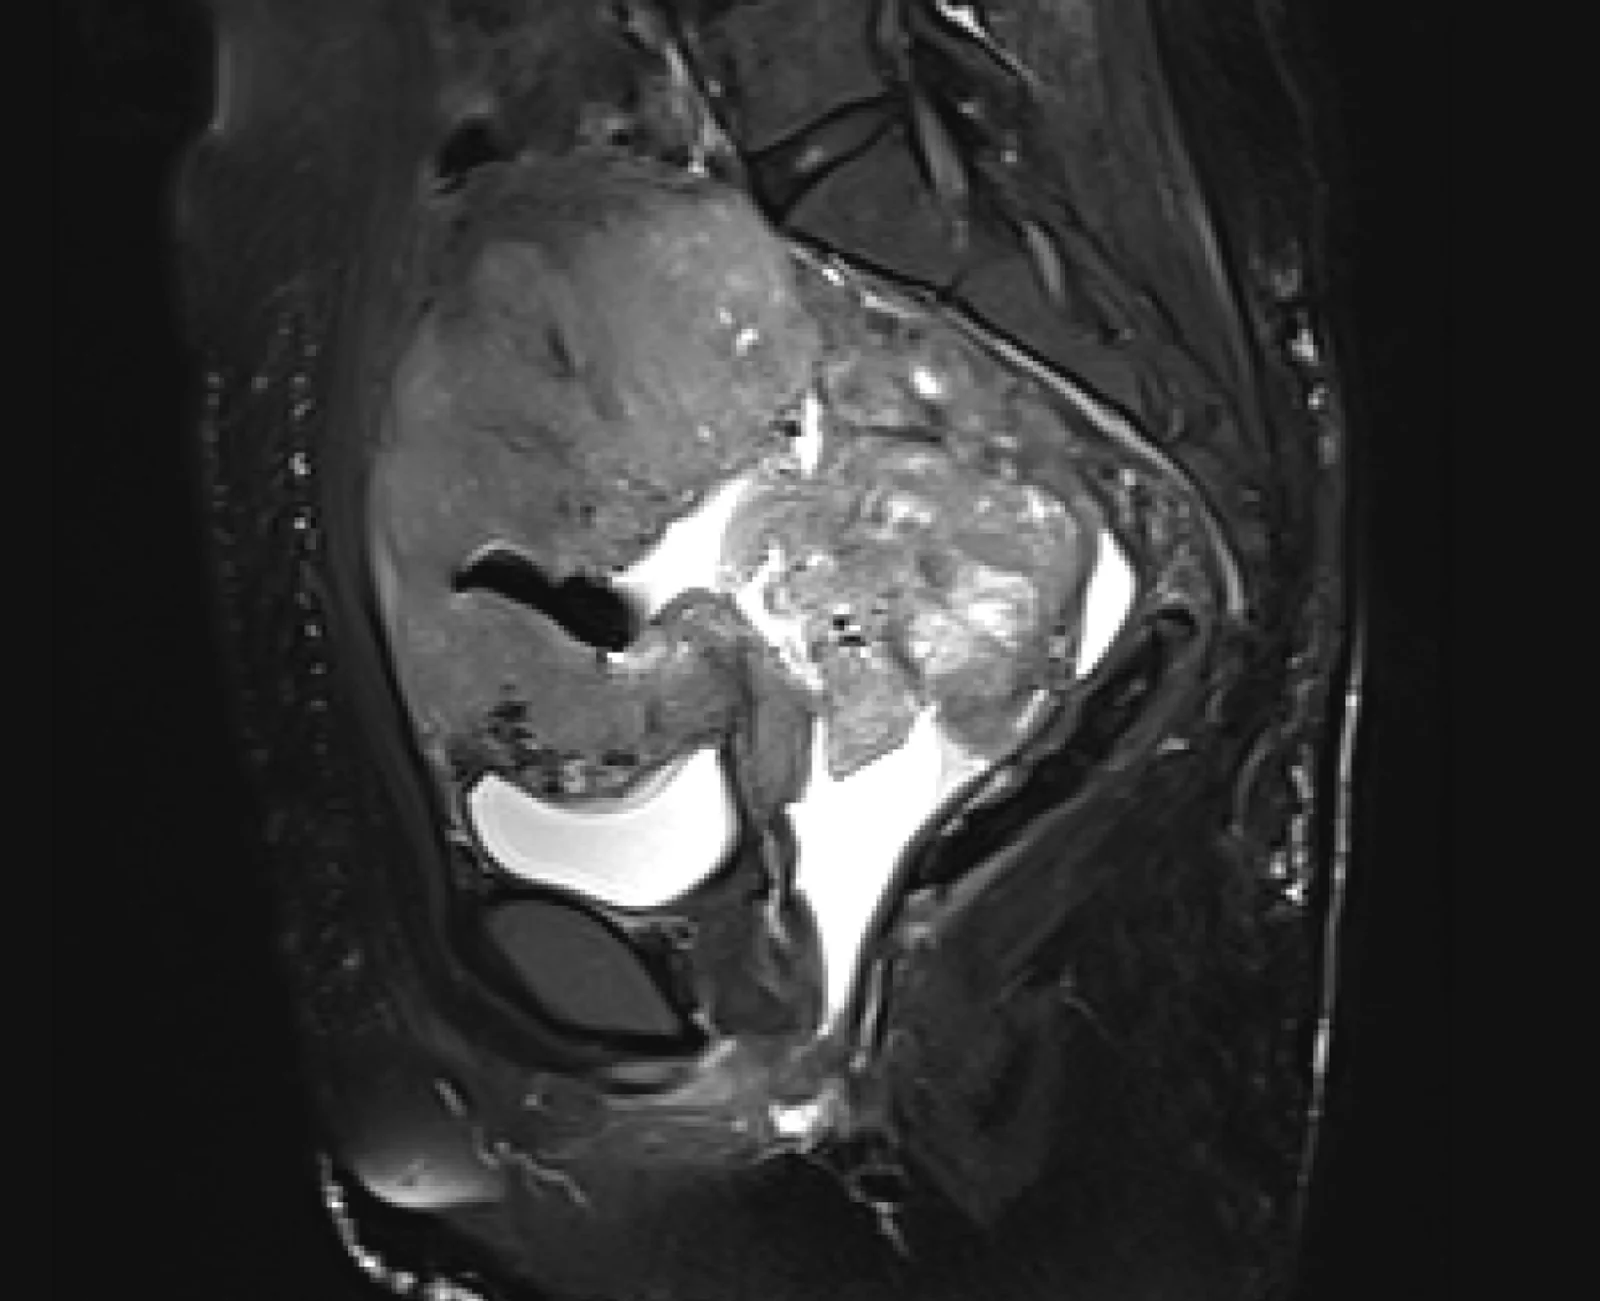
Pretreatment pelvic MRI. Sagittal T2-weighted fat-suppressed sequence acquired before NACT demonstrates a massive heterogeneous cervical mass measuring 14 × 13 × 13 cm (arrows), occupying nearly the entire pelvic cavity, with compression of the lower ureters and bilateral hydronephrosis. MRI = magnetic resonance imaging, NACT = neoadjuvant chemotherapy.
